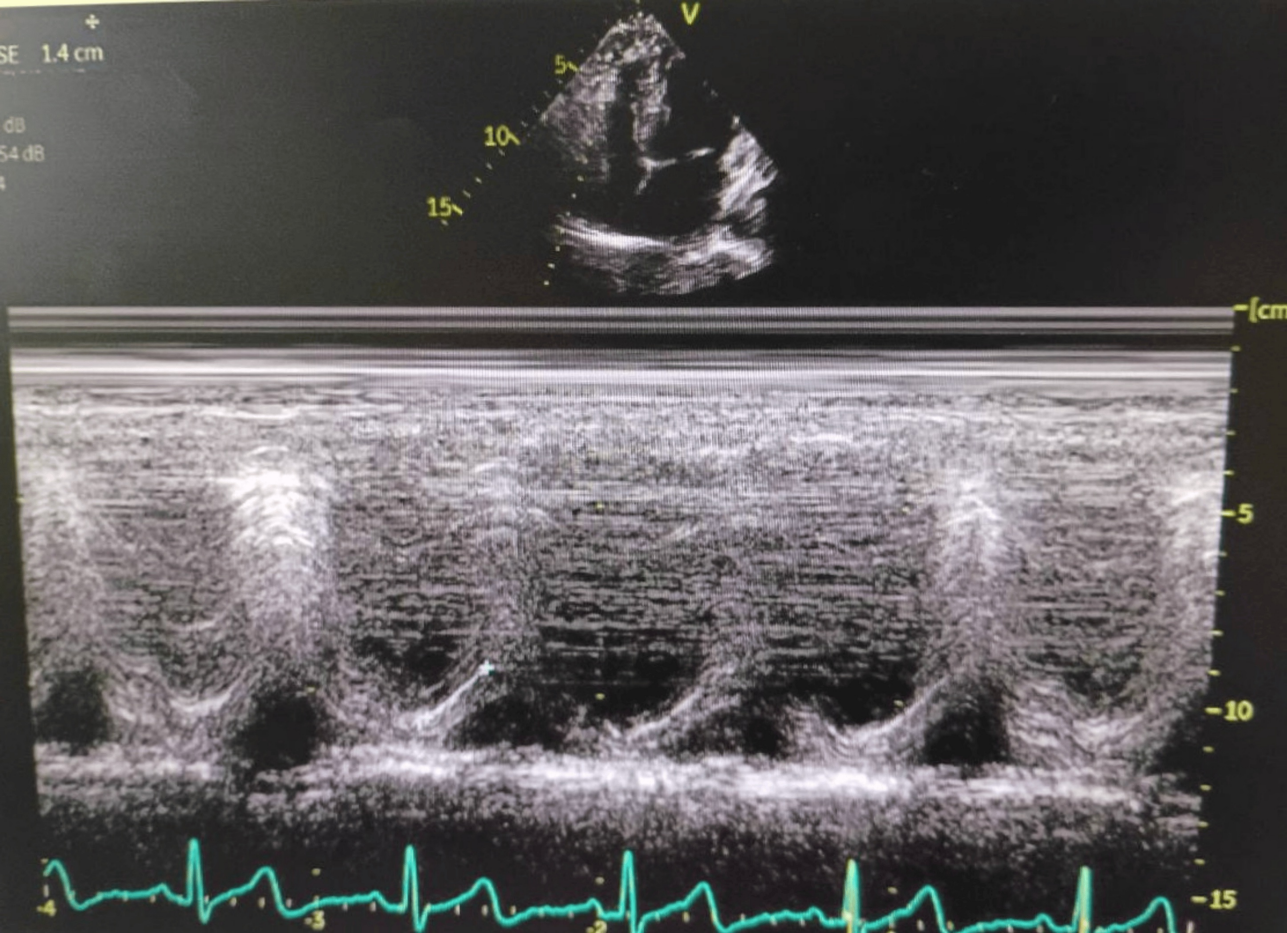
Echocardiogram showing a normal right ventricular function.
